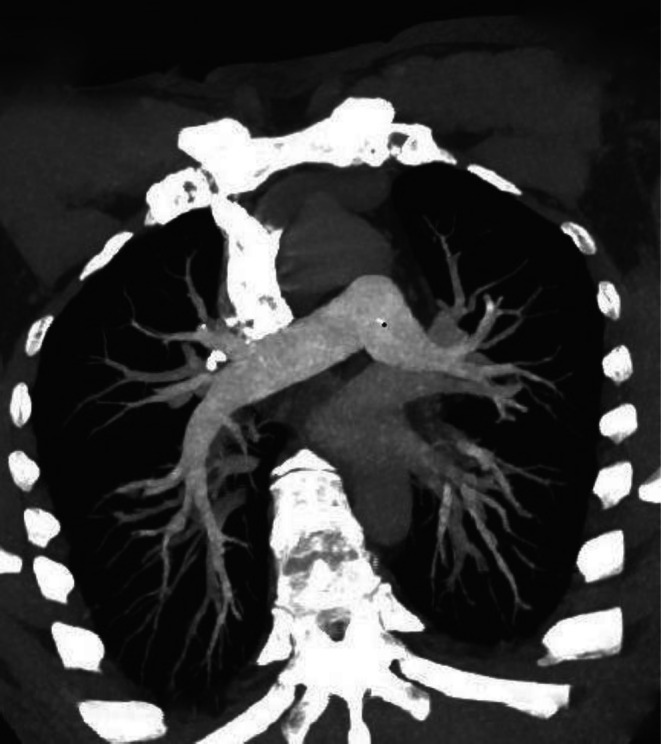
Follow‐up contrast‐enhanced CT pulmonary angiography demonstrating complete resolution of previously detected pulmonary emboli after treatment and clinical recovery.
